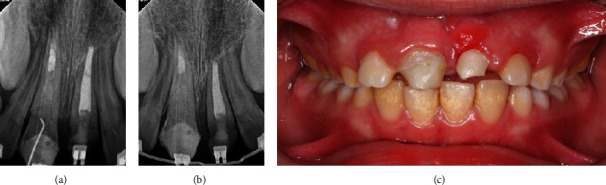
Periapical radiographs showing (a) midway through active orthodontic eruption and (b) after 2-month stabilization before removing the brackets. Tooth #21 shows good crown-to-root ratio after full extrusion. (c) Clinical picture showing the position of teeth after removal of the brackets.
